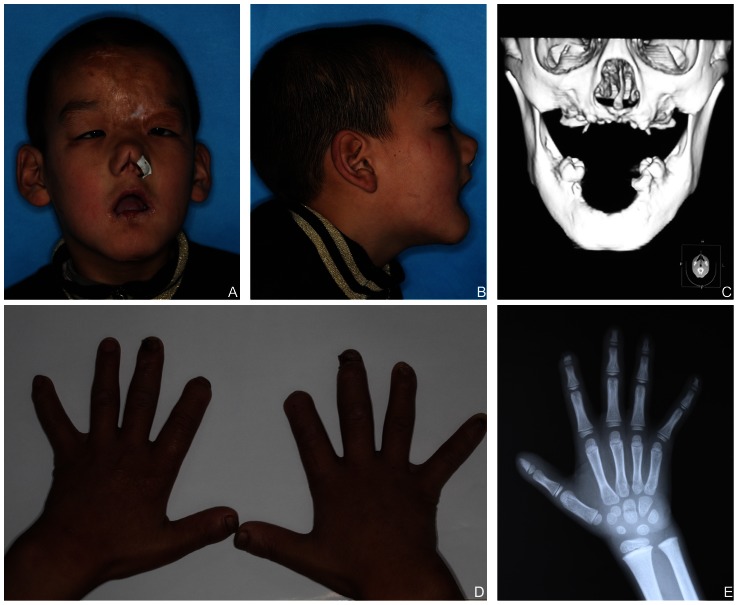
Clinical features of the proband. (A) Scratch marks, erosions, cicatrization healing (scars) due to self-inflicted soft tissue trauma, nasal columella defect and keratitis. (B) Abnormal profile, limitation of mouth opening. (C) A cranial CT scan and reconstruction of head. (D) Lichenification, missing tips of right index fingers, and the deformed nail and left digitus minimus. (E) Radiograph of the hand and wrist bones.
